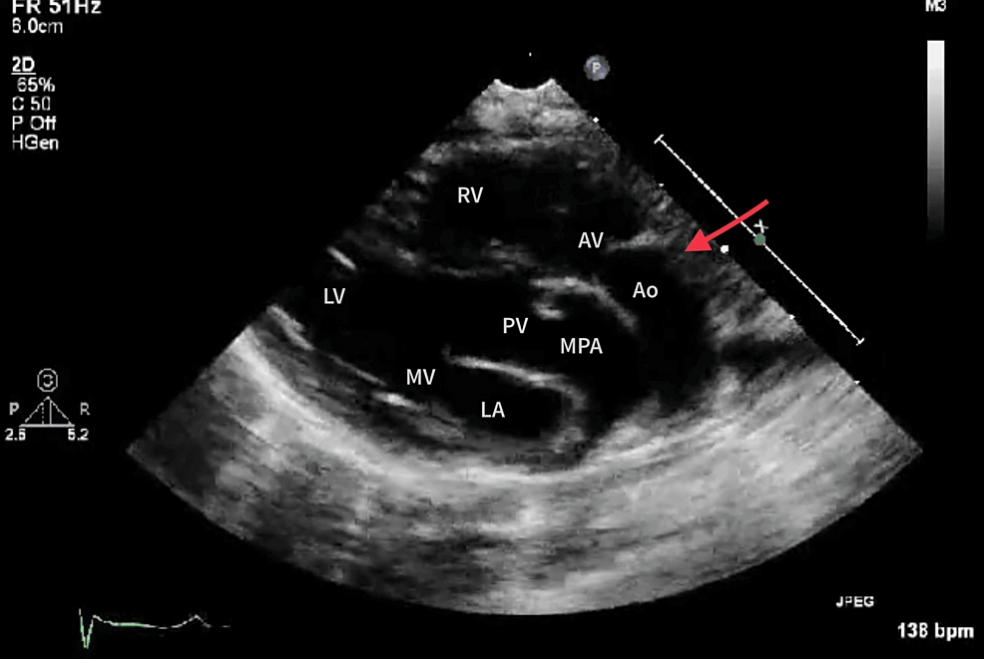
Parasternal long-axis view suggesting transposition of the great arteries Red line showing the aorta originating from the right ventricle. LV, left ventricle; RV, right ventricle; MV, mitral valve; PV, pulmonary valve; LA, left atrium; Ao, aorta; AV, aortic valve; MPA, main pulmonary artery
